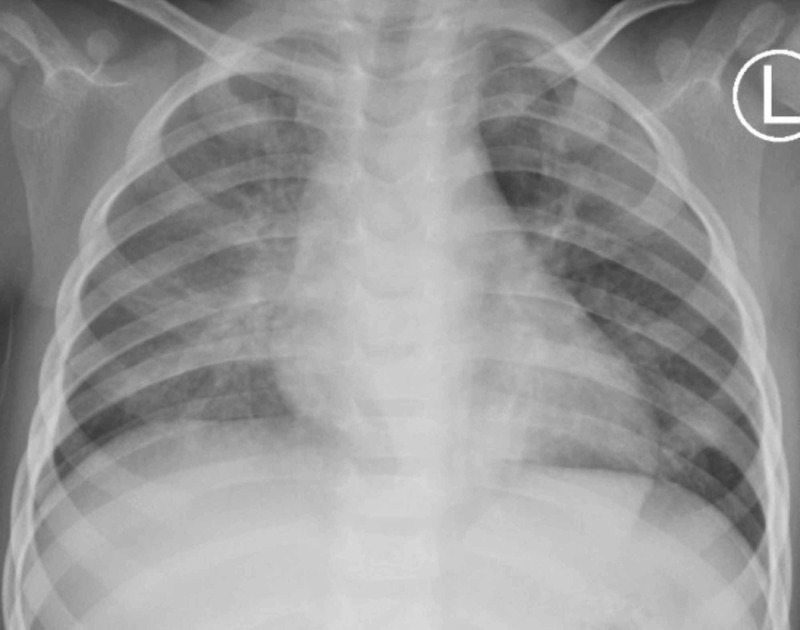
Chest X-ray on first post-operative day demonstrated resolution of pneumothorax
